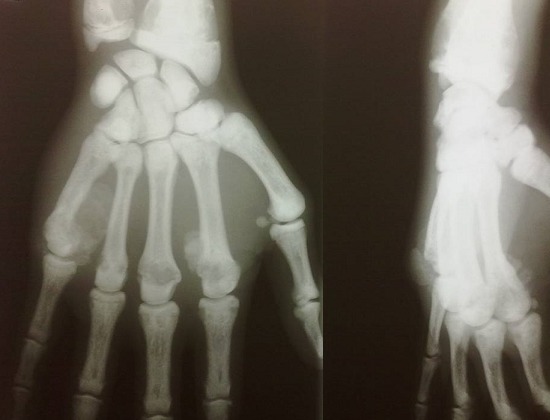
Radiographie des mains prenant les poignets: multiples images lytiques et condensantes
